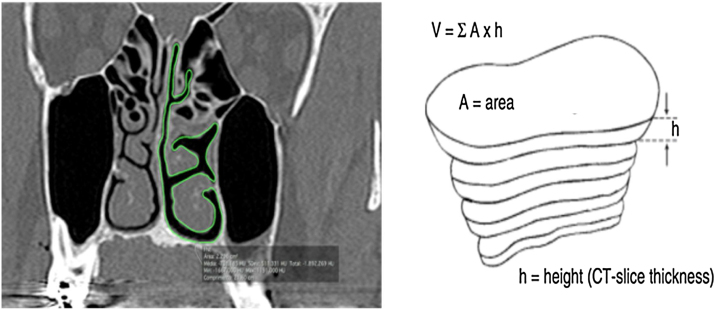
Coronal cut area delimitation and volume reconstruction. (A, CT coronal Cut; B, Reconstruction Technique) (From Rodrigues et al., 2017).^5^.
